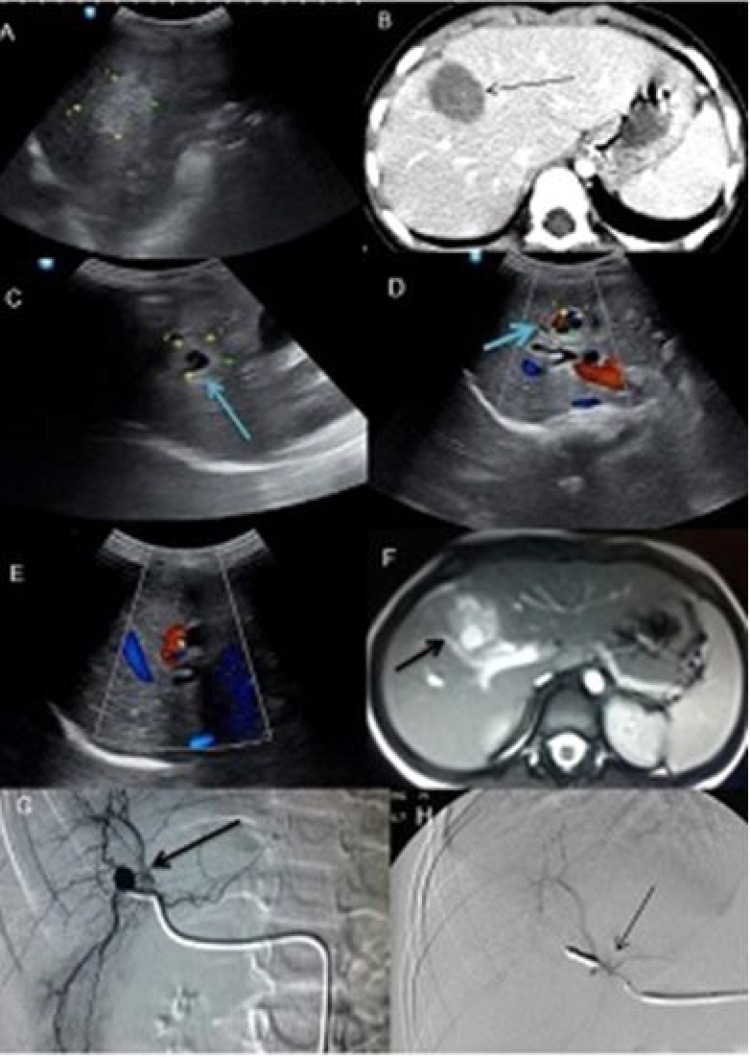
A) Ultrasound showed a hyper echo heterogeneous ill-defined lesion (arrow) in the right lobe of liver measuring 30*40 mm in favor of hematoma. B) Axial contrast-enhanced CT scan correlated with ultrasound finding (arrow). The patient underwent conservative treatment. C, D, E) One month later, ultrasound revealed a 23*23 mm hyper echo lesion with central 14*14 mm hypo echo vascular area in segment 8 of liver (an aneurysmal lesion). F) Axial T2W MRI showed a round hyper signal lesion (arrow), the same signal with aorta. G, H) Selective digital subtraction angiography (DSA) from right hepatic artery shows pseudo-aneurysm in right hepatic artery. The patient underwent coil embolization for treatment of aneurysm (arrow) and aneurysm disappeared after embolization (arrow)
